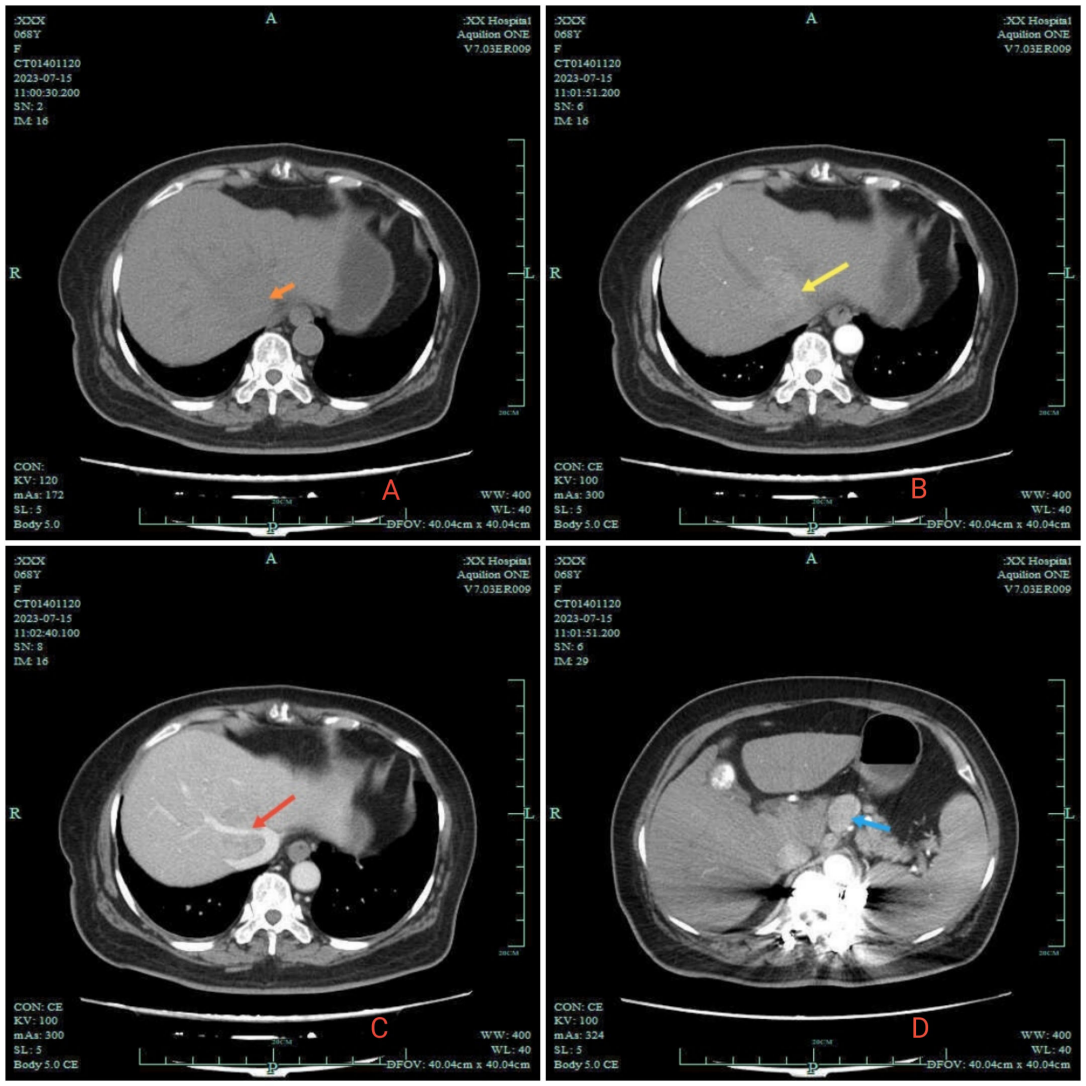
2023–7 Abdomen CT. (A) orange arrow in A indicates the low-density shadow of the clumps. (B) is the enhanced arterial phase: yellow arrow reveals significant enhancement of the tumor. (C) is the enhanced venous phase: red arrow shows the middle hepatic vein wrapped in the shadow of the mass. Enlarged lymph nodes are indicated by blue arrows in (D).
